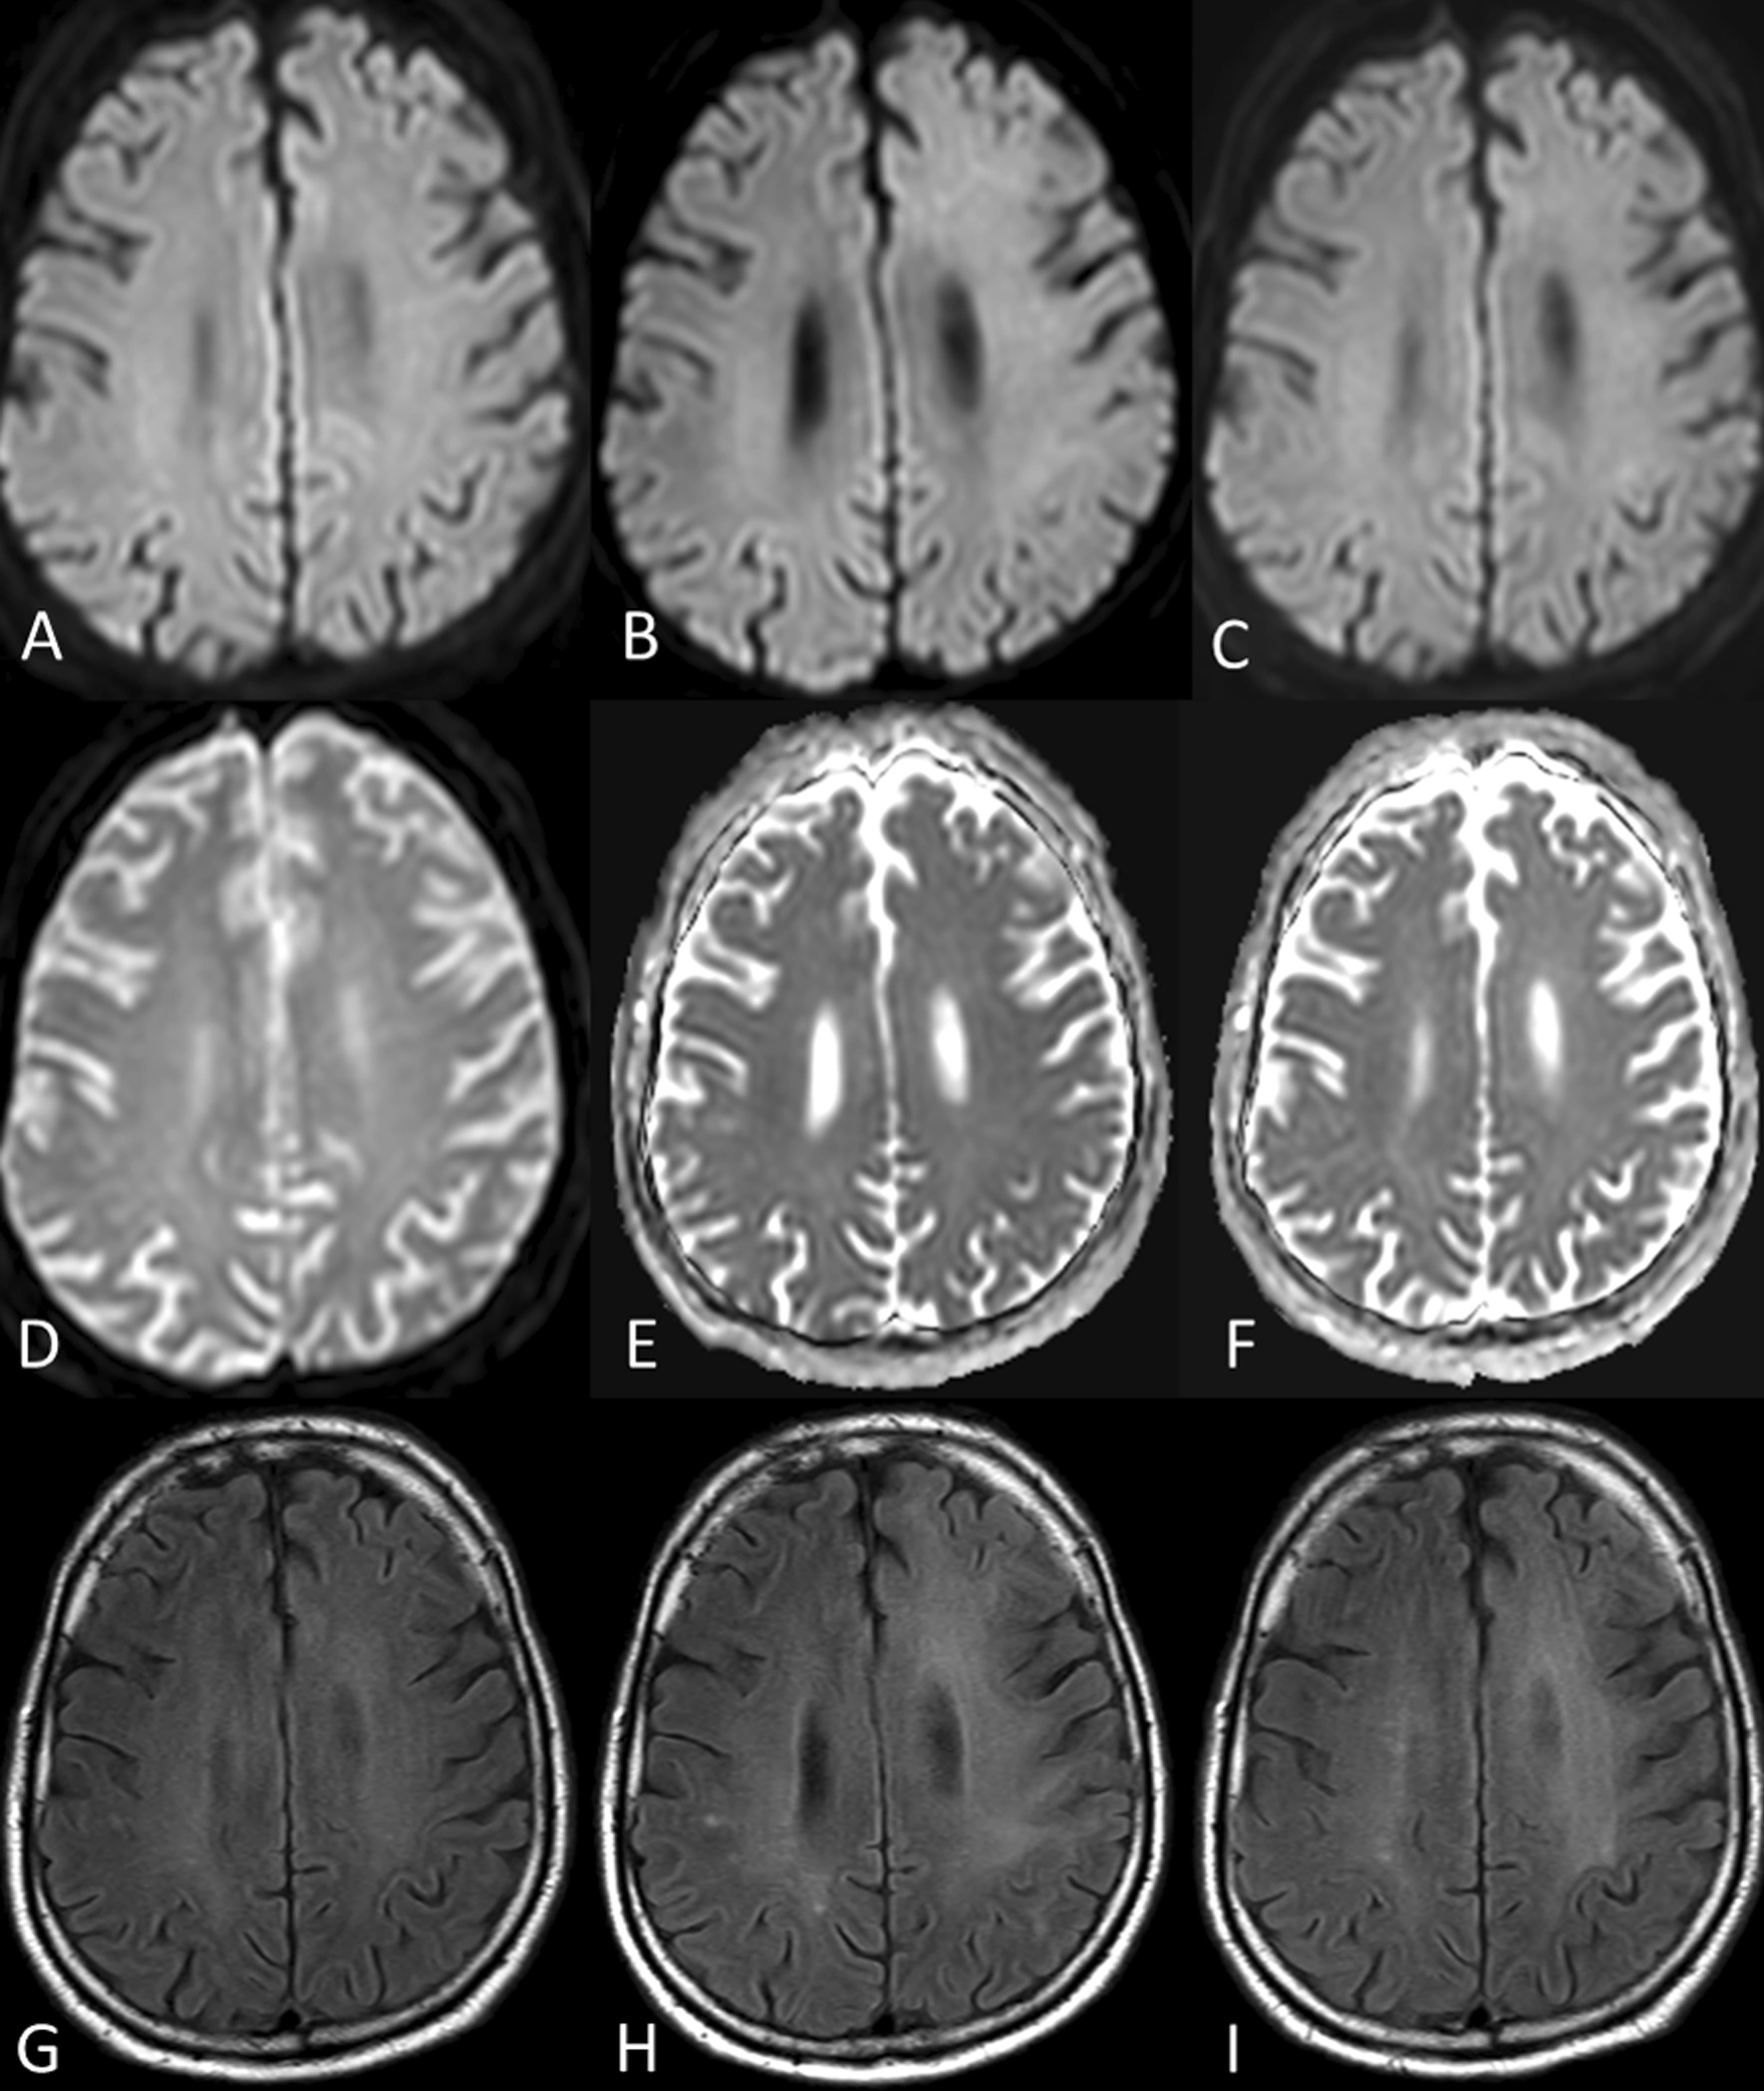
Diffusion-weighted imaging (DWI, top row), apparent diffusion coefficient (A, D, C, middle row) and fluid attenuated inversion recovery (FLAIR, bottom row) imaging at day 10 (A, D and G), day 23 (B, E and H), and day 92 (C, F and I) after endovascular recanalization of a left internal carotid artery occlusion. Please note the homogeneous white matter hyperintensity in the left anterior and middle cerebral artery territory on FLAIR images, which is not visible on day 10 (G), but on day 23 (H) and also on day 92 (I), sparing the subcortical U-fibers and with inconspicuous DWI at each corresponding MRI
